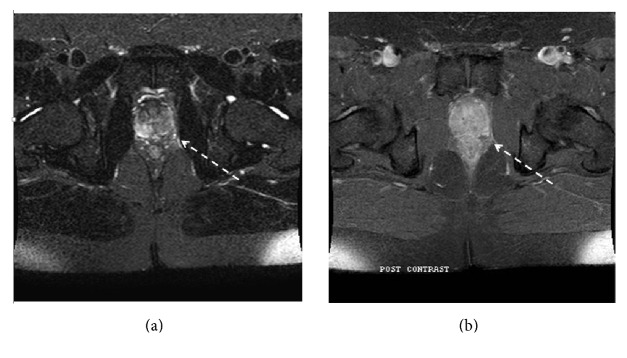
MRI of pelvis: axial FS T2WI (a), axial postcontrast T1WI (b). After aspiration and antibiotic therapy showing resolution of the previous intraprostatic collection.
